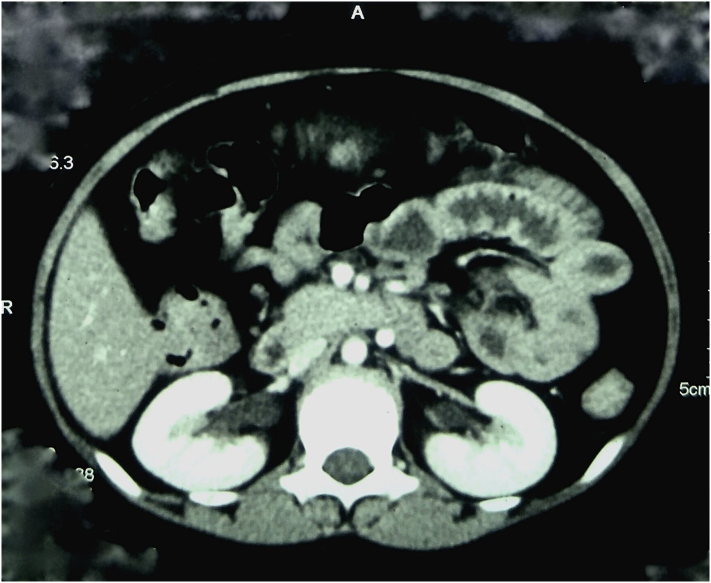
CT abdomen showing multiple heterogeneously enhancing polypoidal endophytic soft tissue in the rectum, sigmoid colon, descending colon, mid aspect of the transverse colon, and ascending colon.
